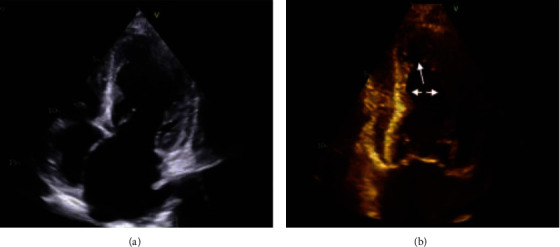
Transthoracic echocardiogram four-chamber view (diastolic image on (a), systolic image on (b)) demonstrating classic pattern of apical akinesis and hypercontractility of midbasal segments of the LV (arrows on (b) emphasizing this specific pattern of wall motion abnormalities seen in Takotsubo's).
